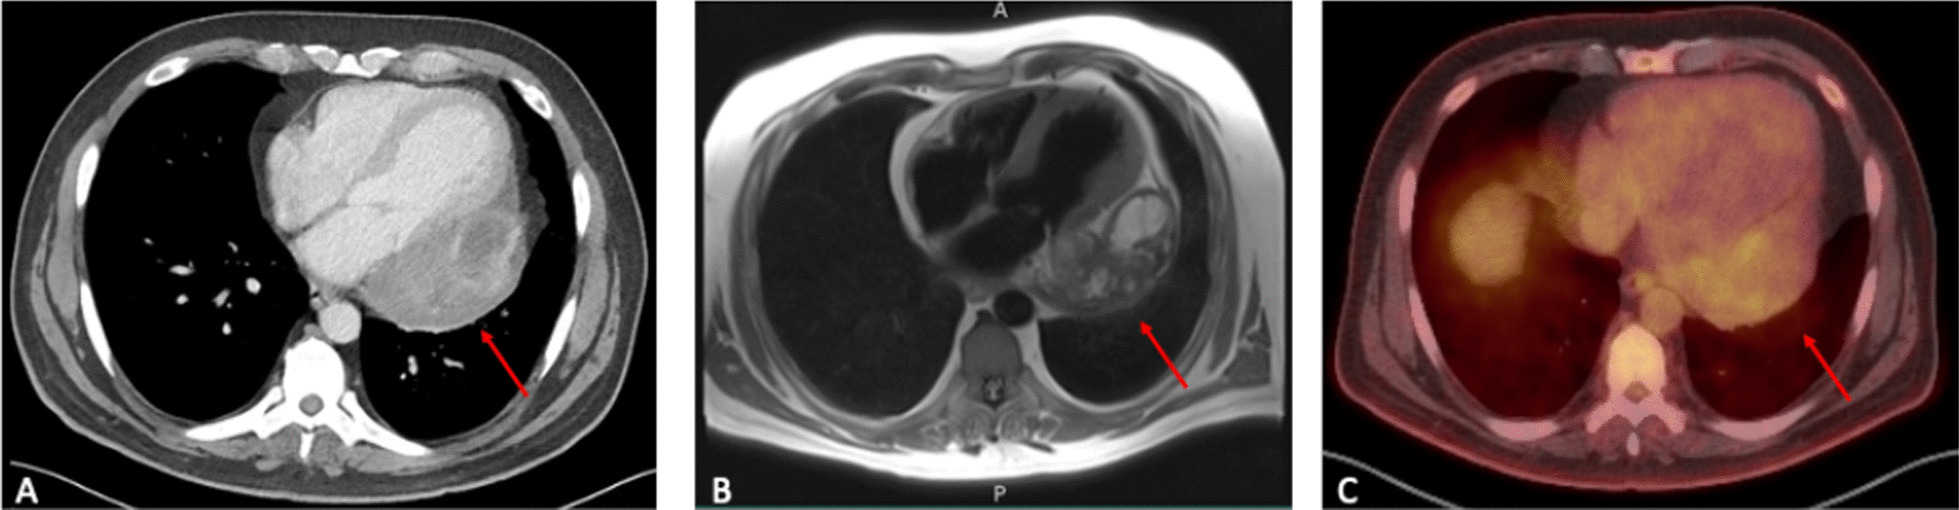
A CT scan showing a lesion along the left aspect of the pericardium. B MRI confirming the presence of a predominantly T1 isointense, mildly enhancing mass abutting the left atrium, left ventricle, and left pulmonary veins. C PET scan demonstrating a mass with mild heterogeneous FDG uptake
